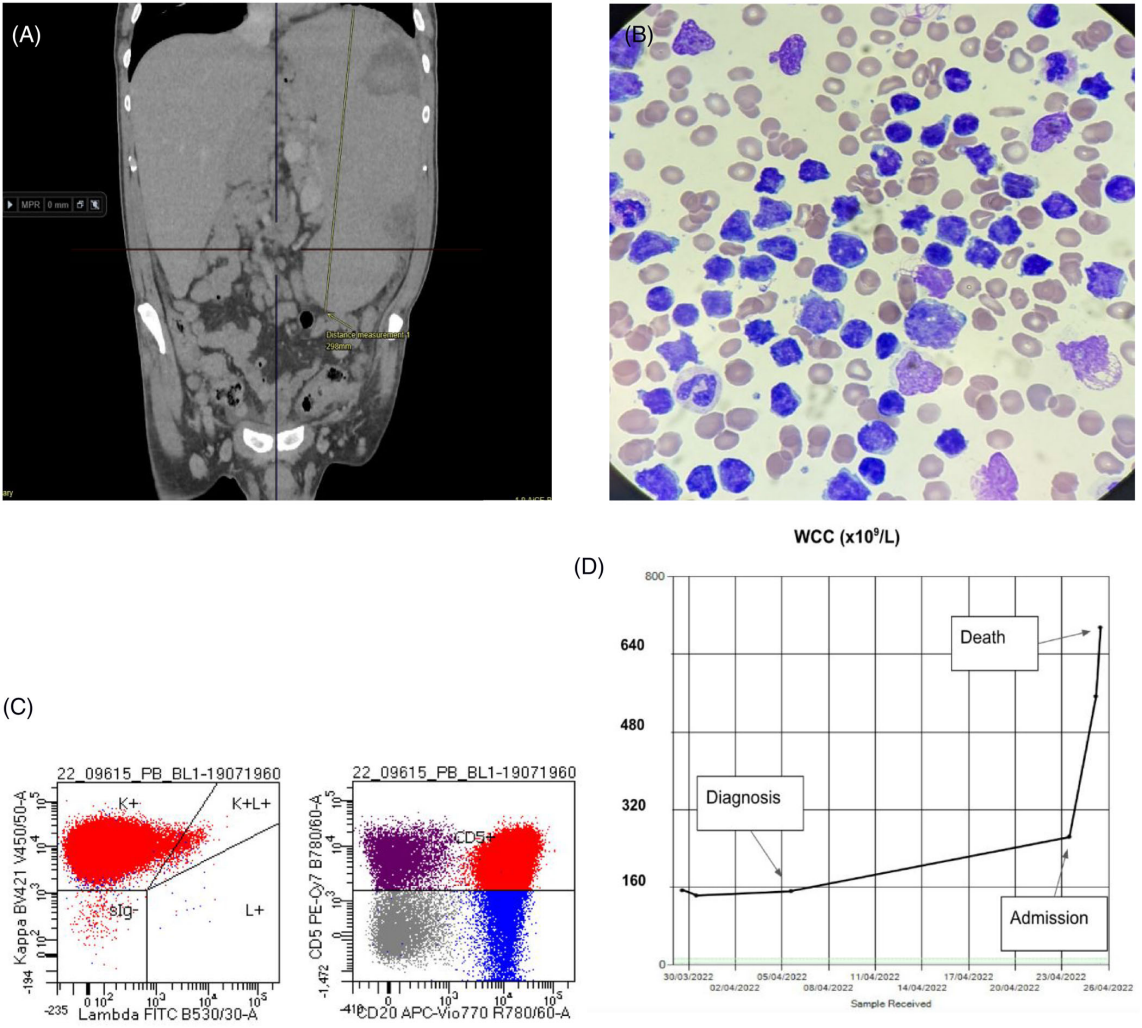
(A) CT demonstrated massive splenomegaly measuring 30 cm at maximum diameter. (B) Pleomorphic abnormal lymphocytes with condensed chromatin and irregular nuclei. (C) Flow cytometry demonstrated a kappa light chain restricted population of CD20 and CD5 positive lymphocytes. (D) Explosive increase in WBC count coincided with the onset of spontaneous tumour lysis syndrome.
